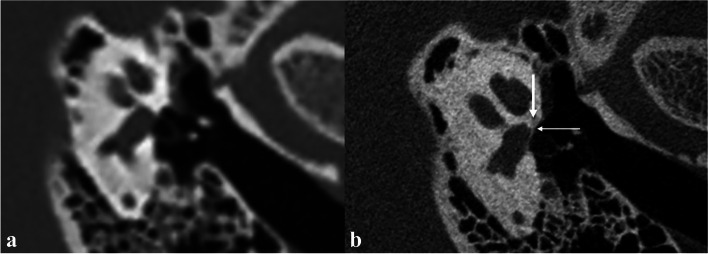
Typical imaging findings of fenestral otosclerosis in the otosclerotic phase. HRCT (a) shows no definite positive signs. U-HRCT (b) shows no obvious reduction in the density of fissula ante fenestram (thick arrow), increased density of adjacent annular ligament, and slightly thick stapes footplate (fine arrow)
